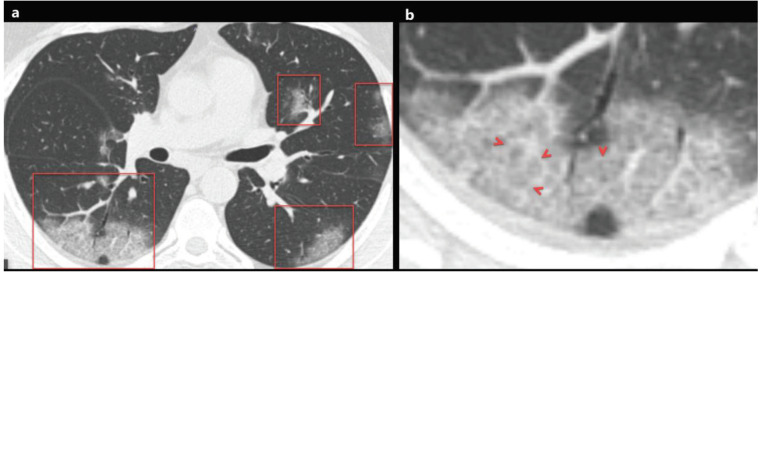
a) A 48-year-old man with COVID-19 presented with cough, fever, myalgia, and malaise for 3 days. Axial chest CT image shows multifocal, peripheral-peribronchovascular ground-glass opacities (red frames). b) The magnified image of the right lower lobe shows ground-glass opacity superimposed with interlobular septal thickening and prominent intralobular lines (red arrowheads), which indicates a crazy-paving pattern.
